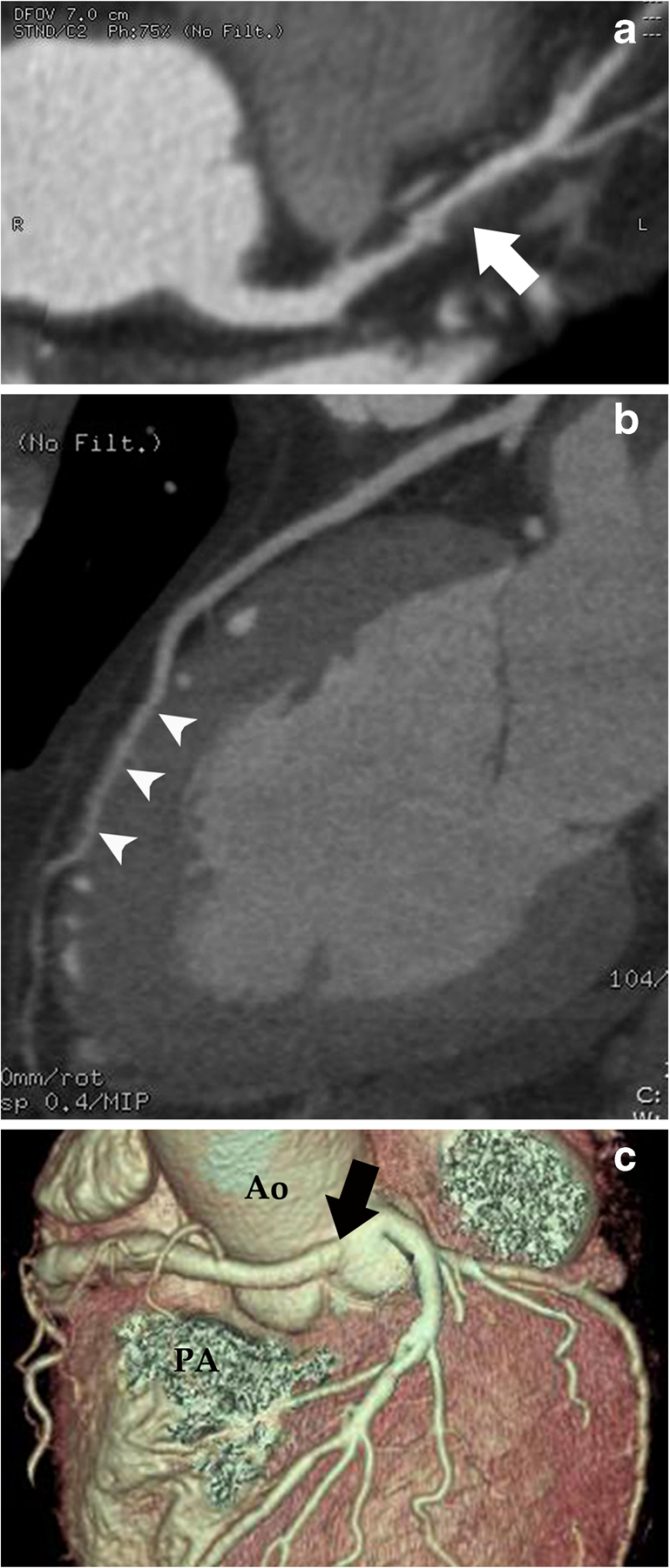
Different kinds of computed tomography coronary angiography (CTCA) images showing examples of coronary diseases. a Transverse curved planar reformatted (CPR) image showing a non-calcified plaque in the mid-segment of the left anterior descending coronary artery (*arrow*), causing a significant (> 50%) stenosis. b CPR image along the left ventricle vertical long axis showing a 3-cm-long myocardial bridging (*arrowheads*) of the mid-distal third of the left anterior descending coronary artery. c Three-dimensional volume rendering image showing the origin from the left Valsalva sinus of the right coronary artery (*arrow*), which reaches the right atrium-ventricular path, coursing between the aorta root (*Ao*) and the pulmonary artery root (cut – *PA*) (malignant course)
